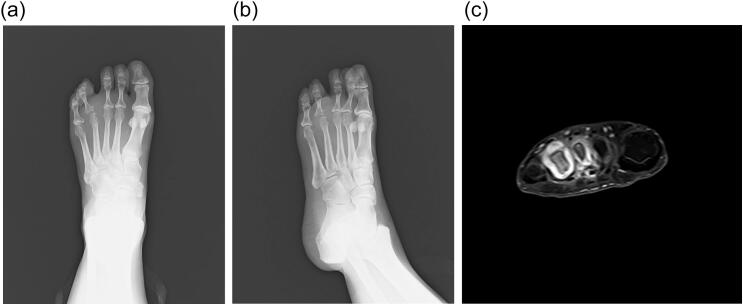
Foot involvement in a 50-year-old female. Anteroposterior (a) and oblique (b) plain radiographs of the left foot. Axial T1-weighted fat suppressed contrast enhances MRI image (c) showed synovial proliferation and fluid around 3rd and 4th metatarsals.
